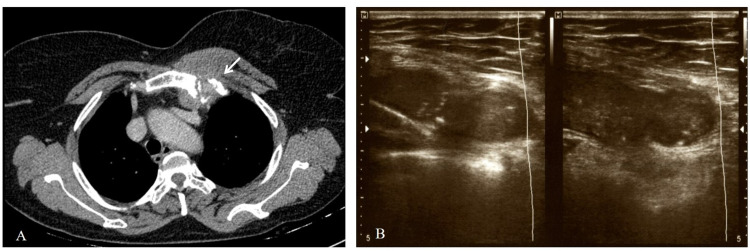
(A) CT scan of neck region showing (arrow) the necrotic destruction of left sternoclavicular joint with involvement of adjacent soft tissue. (B) USG showing the fluid-filled swelling with a needle in situ for aspiration.
